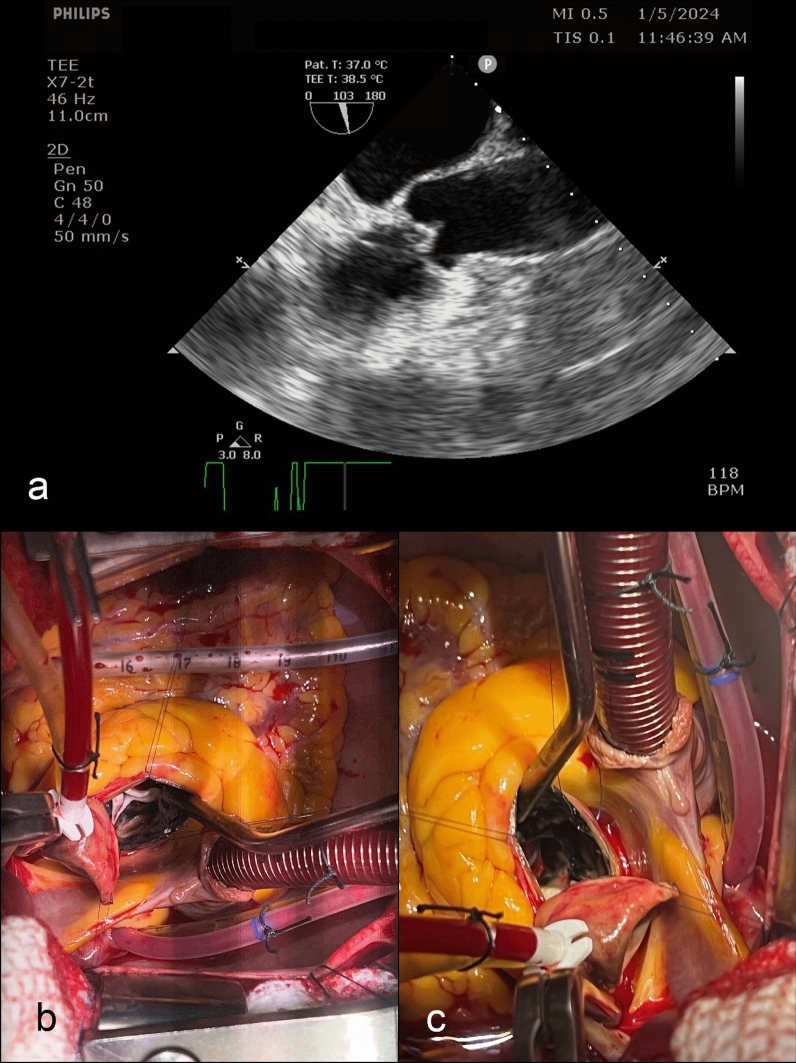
a Tranoesophageal echocardiography (TEE) showing no evidence of aortic dissection. Intraoperative findings of the patient. b and c black pigmentation extending to the aortic valve leaflets and the anterior mitral leaflet (AML) base
